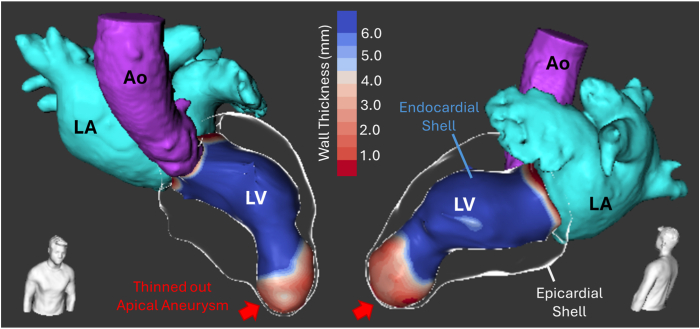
Pre-procedural wall thickness CT model (visualized with Adas3D, Galgo Medical). Ao = aorta; CT = computed tomography; LA = left atrial; LV = left ventricular.
